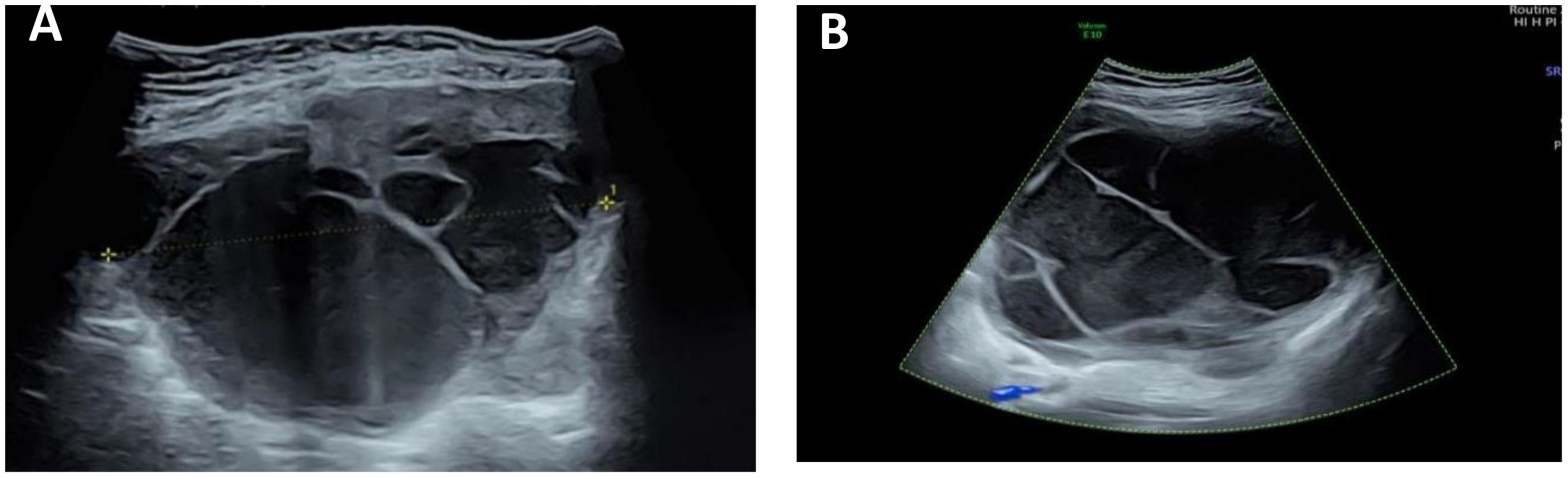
Ultrasound image of the tumor. (A) There is a separation in the mass, and the wall thickness is not smooth. (B) A weak light spot is seen in the mass, and there is no blood flow signal in the mass.
